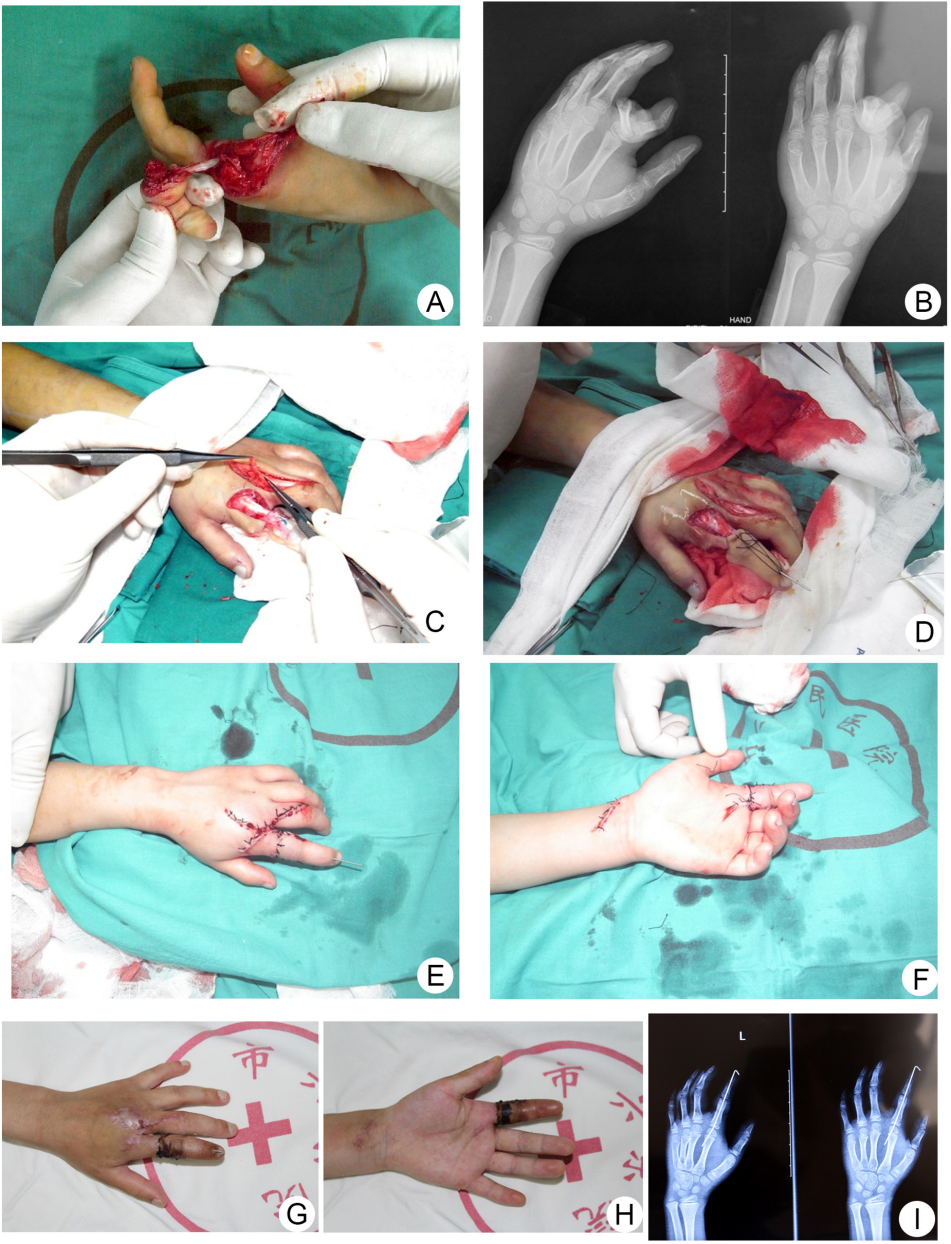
Emergency finger replantation surgery after COVID-19 virus nucleic acid testing normalization. (A,B) Preoperative appearance and radiography. (C–F) Intraoperative appearance. (G–I) Appearance and radiography 1 month after surgery. COVID-19, corona virus disease 2019.
